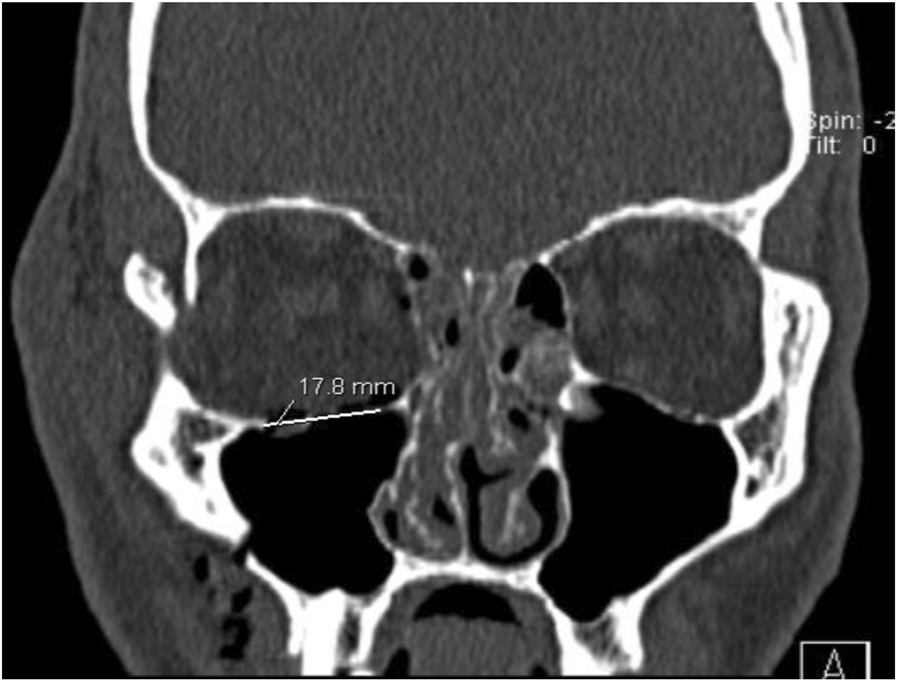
Coronal slice of a native CT-scan of the midfacial area for the measurement of orbital floor defect sizes. Shown is a zygomatic bone fracture on the right side involving the lateroorbital column, the orbital floor and the anterior maxillary sinus wall
